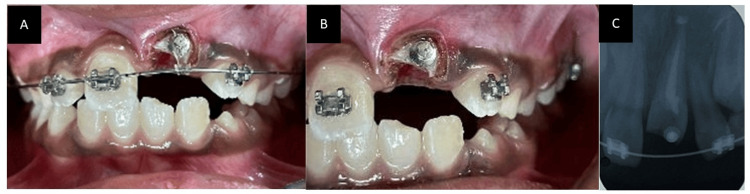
Orthodontic extrusion phase. A & B) Intraoral frontal views showing the application of continuous orthodontic traction with a segmental fixed appliance (MBT, 0.022" slot) and a 0.016" NiTi archwire, using elastomeric traction. Adjacent maxillary anterior teeth serve as anchorage units. C) Periapical radiograph during the extrusion phase confirming progressive coronal repositioning of the intruded incisor within the alveolar socket, with maintained root integrity and a developing periodontal ligament space.
